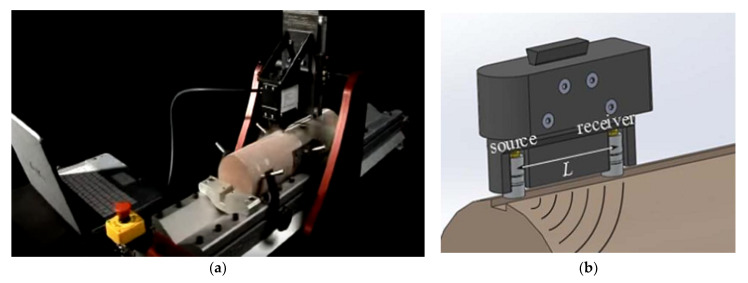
Scratch-test machine used in the study: (a) unconfined compressive strength (UCS) evaluation; (b) ultrasonic velocity acquisition.
